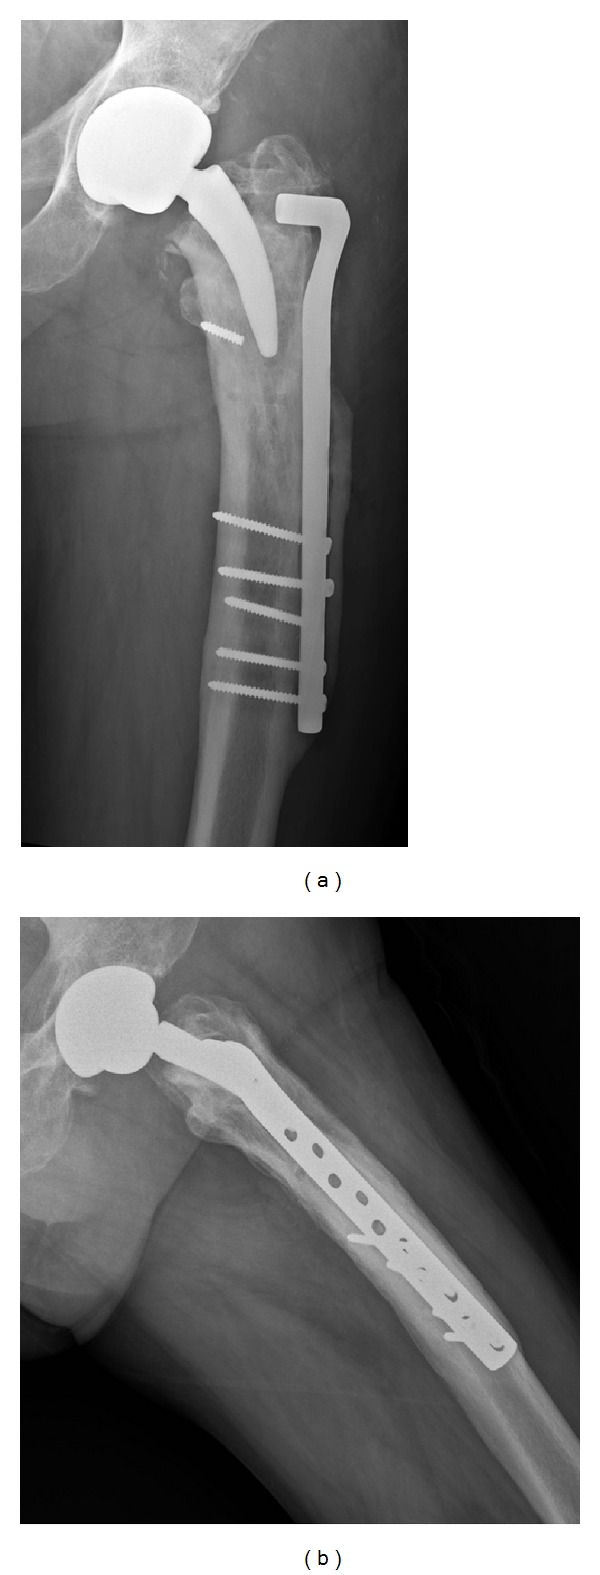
Anteroposterior (a) and lateral (b) radiographs of the left hip one year after the conversion to a short-stemmed THA.
